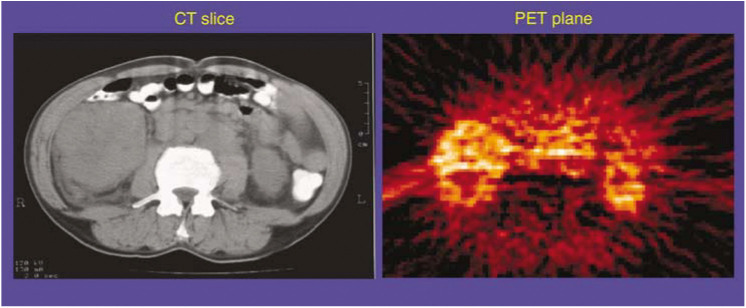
A single CT slice across the abdomen on the left and a single slice from a summed PET H_2_^15^O image on the right. Areas of increased brightness on the PET scan represent increased perfusion (the whiter the area, the higher the perfusion). There is a large right renal mass representing a primary renal cell carcinoma. This is seen on the PET image as the brighter area with a black centre representing a necrotic core. Vascular structures centrally, such as the aorta, also are bright.
